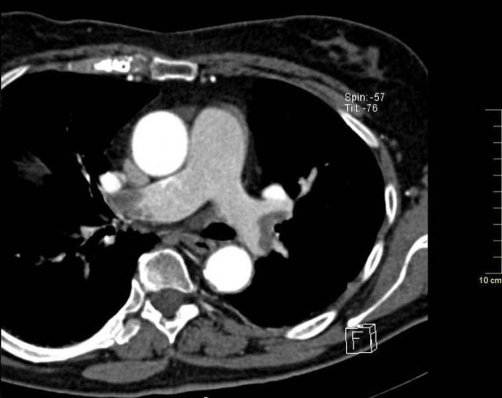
Contrast-enhanced CT image showing massive thrombosis of the right and left pulmonary artery (arrows).
